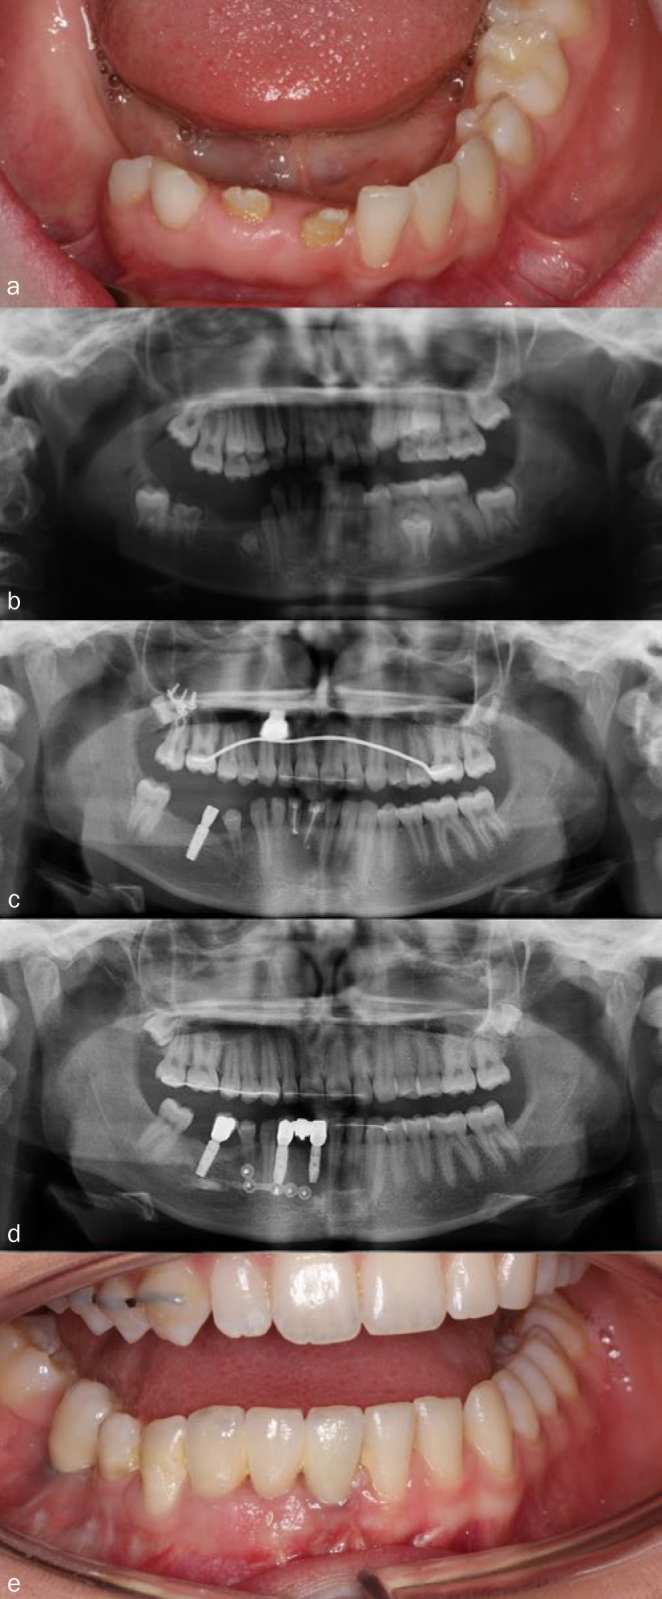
Treatment of patient D, followed for 10 years (from 8 to 18 years of age). a) Clinical photo at age 12. Teeth 42 and 41 were yellow, pitted and irregular shaped, 83 and 43 are present. b) Orthopantomogram at age 11. Radiologically, teeth 46, 44, 42 and 41 have an unusual shape, small in size with large pulp chambers. In addition, agenesis of 45. Note supraposition of teeth in 1st maxillary quadrant. c) Orthopantomogram at age 14. Teeth 44 and 43 have erupted between affected teeth in the region. An implant was inserted region 45. Endodontic treatment of 41 and 42. d) Orthopantomogram at age 18. 41, 42, 83 extracted. Two implants, inter positional sandwich osteotomy, to level the right side of the mandibular occlusal plane. e) Clinical photo of the teeth with crown and the 3-unit bridge in place.
